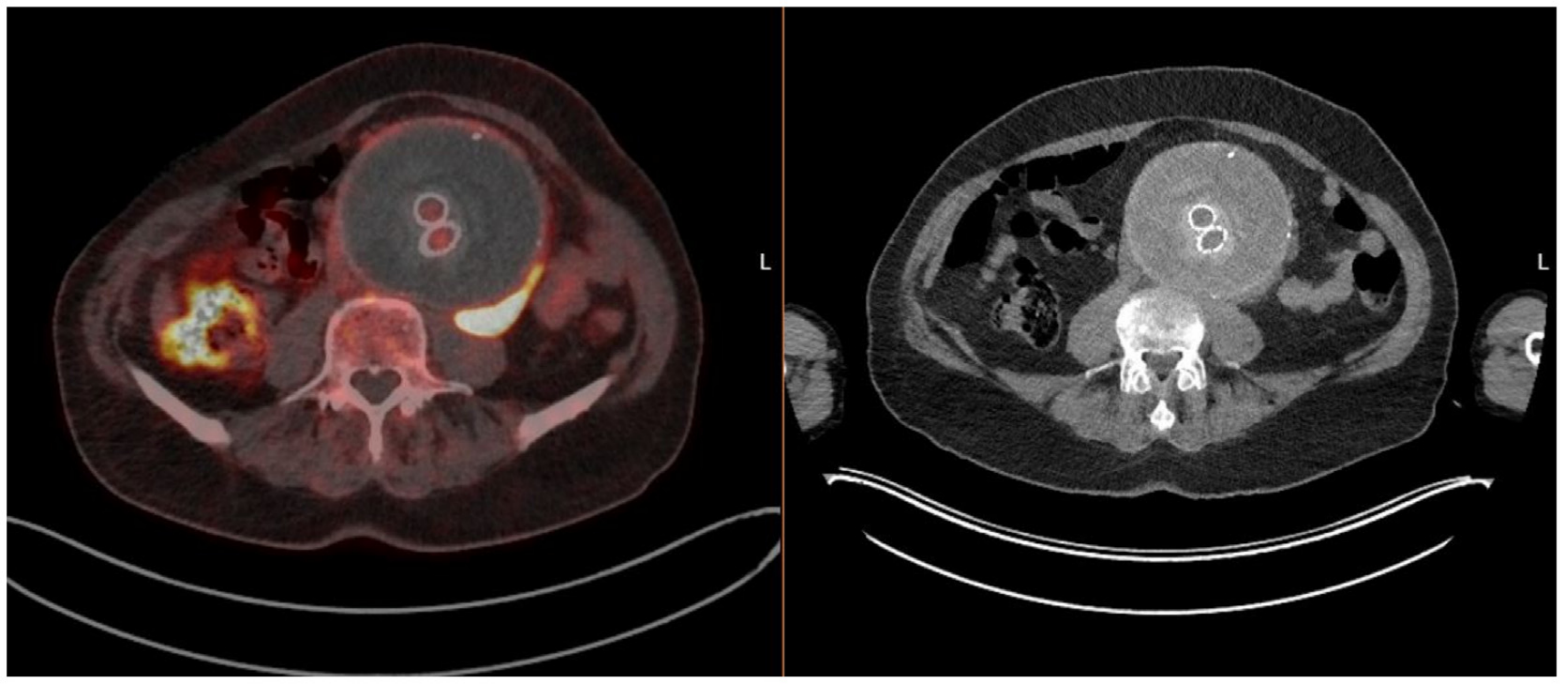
Initial positron emission tomography (PET)-computed tomography (CT) in the referring hospital showing fluid visible on the left lateral side of the aneurysm sac and increased ^18^fluorodeoxyglucose (FDG) uptake.
